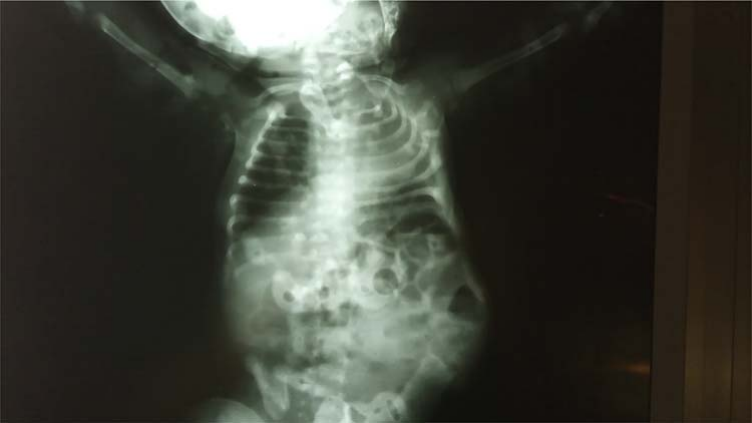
X-ray film shows absence of ribs and mild lumbar scoliosis.
